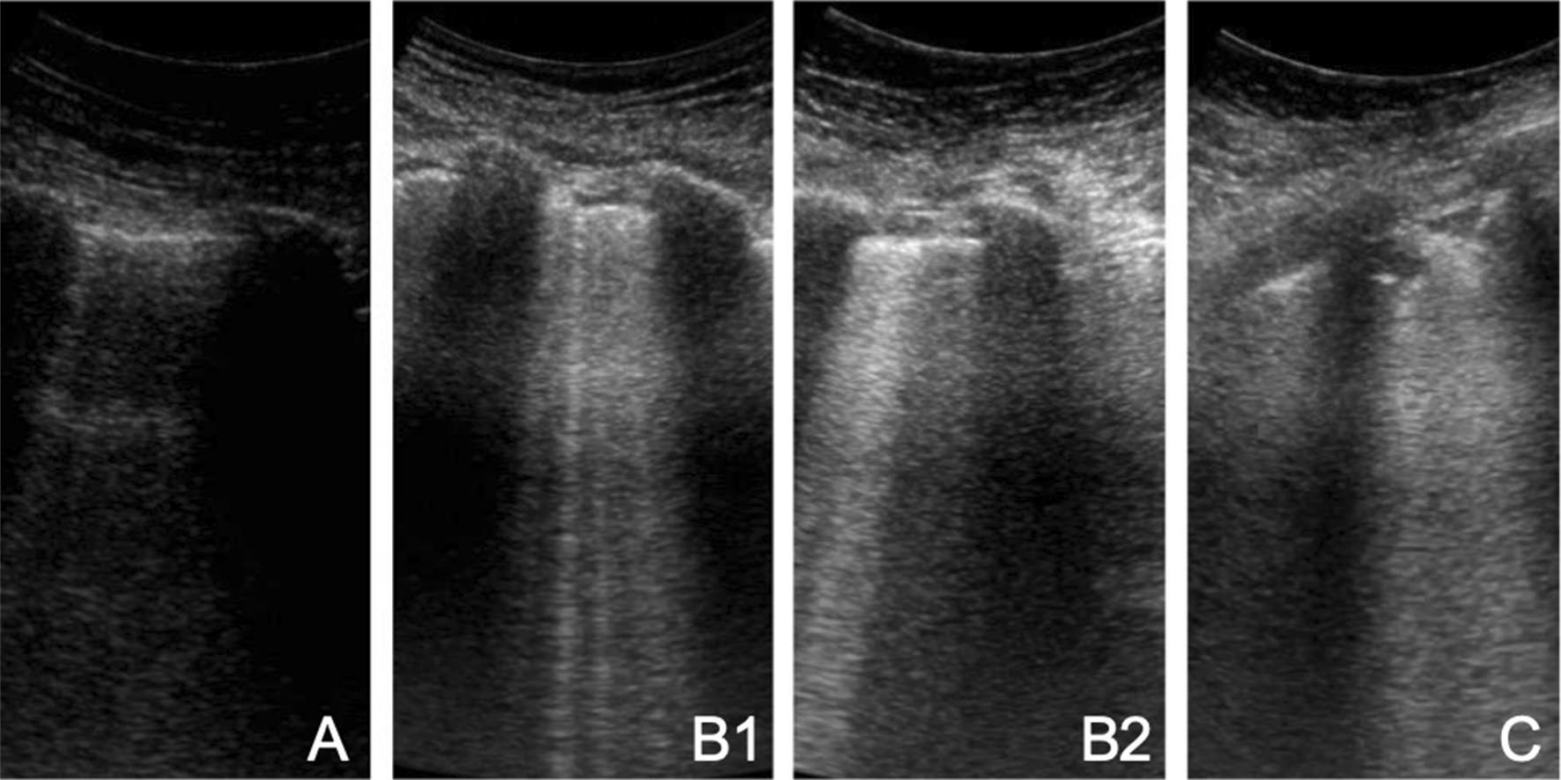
Common Lung Ultrasound patterns in COVID-19 patients. From left to right: ( a, b1, b2, c) pattern
